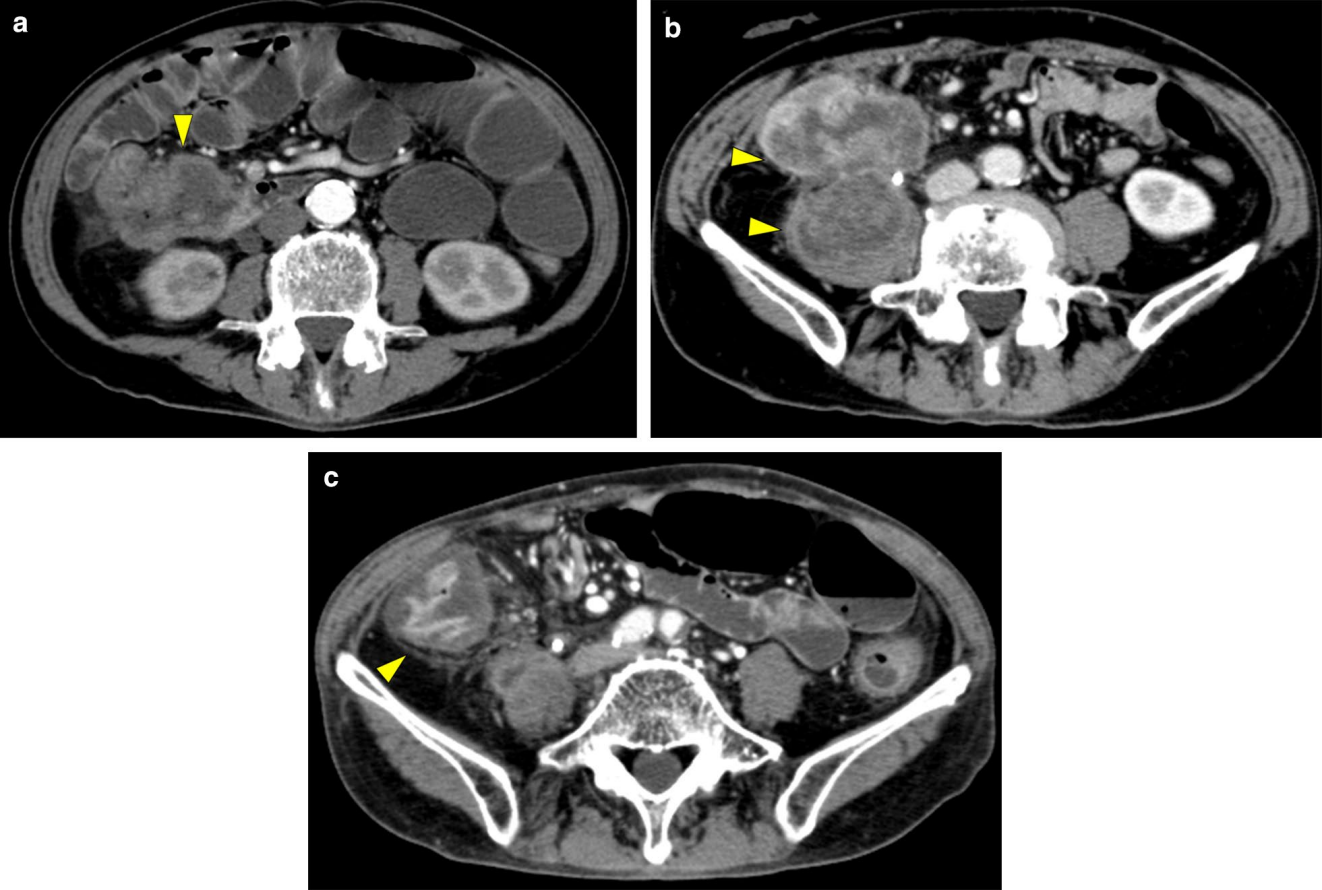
Abdominal contrast-enhanced CT. A 80-mm bulky mass with markedly swollen regional lymph nodes (a), and after six courses of immunotherapy (b), and after radiation therapy (c)
